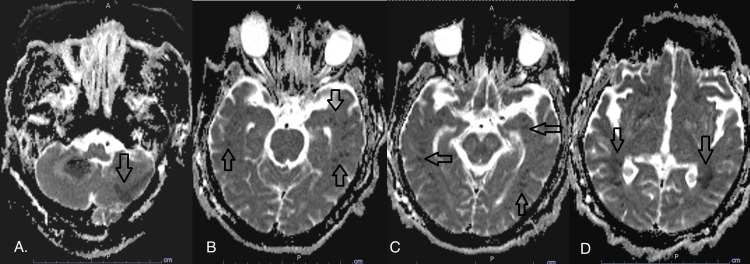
MRI brain diffusion-ADC window MRI brain diffusion-apparent diffusion coefficient (ADC) window showing new left-sided cerebella stroke (arrow in A), with the progression of cerebral strokes (arrows in B, C, and D)
